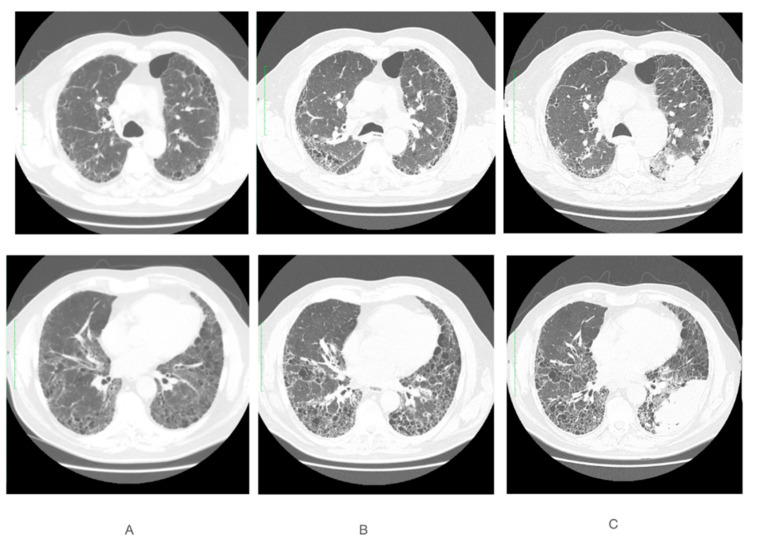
CT scans performed at two depths (upper and lower views) at different time points: (A) November 2019, (B) March 2021 and (C) February 2022.
